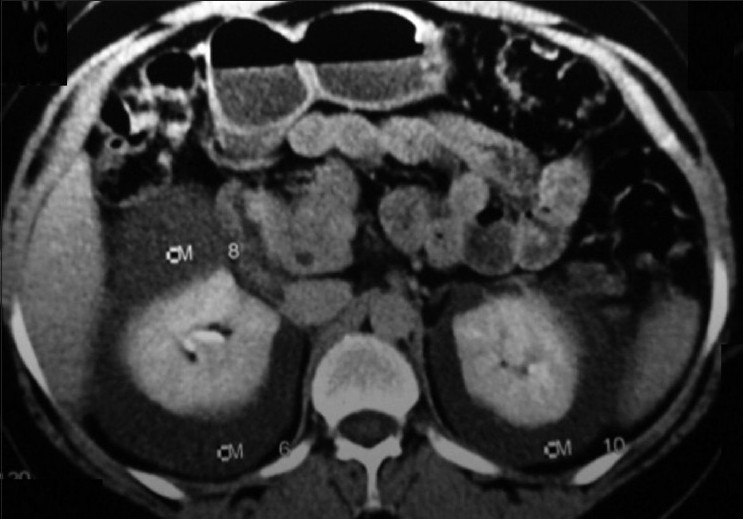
Axial CT scan showing fluid attenuation perinephric collections
